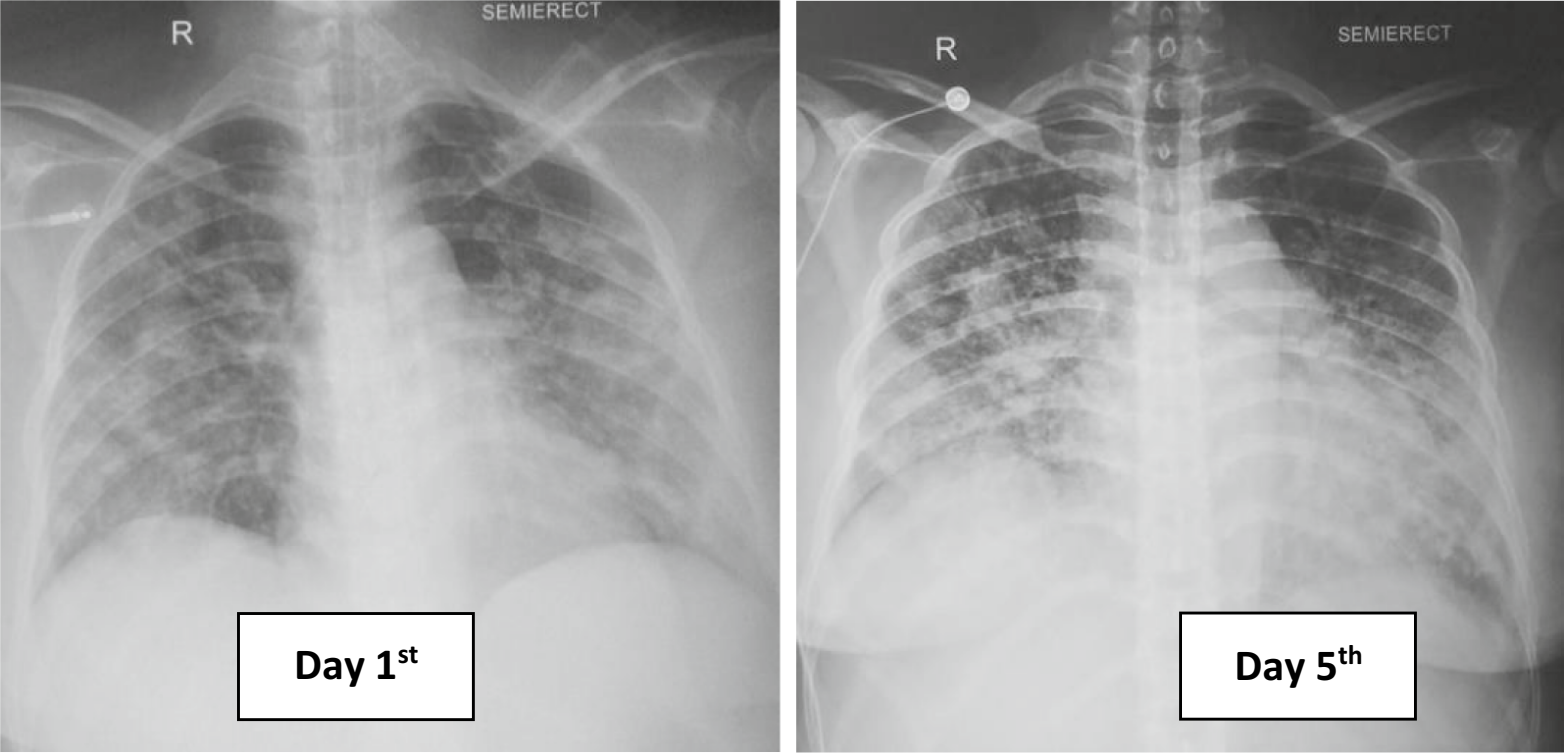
Comparison of chest X-ray within the treatment
